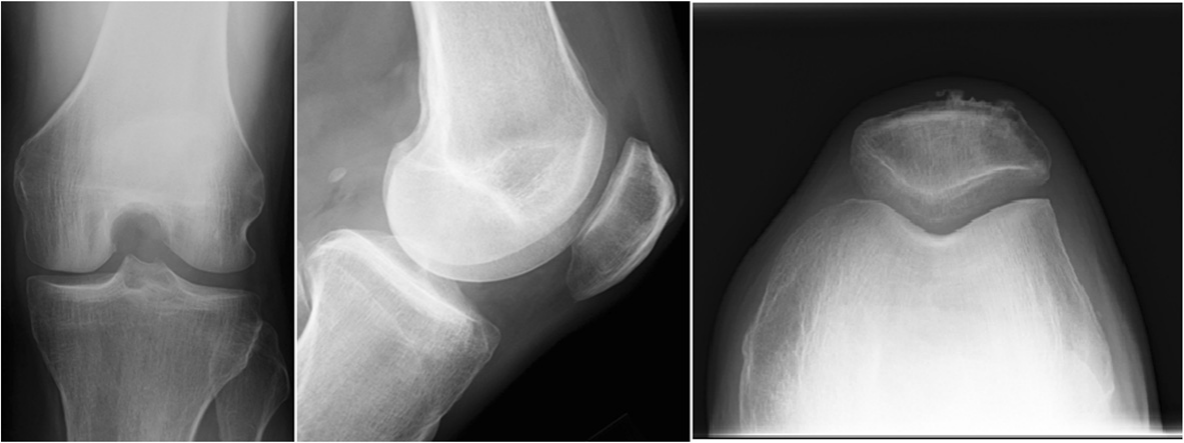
AP, lateral, and sunrise radiographs of the knee demonstrate a lucent lesion of the superolateral patella.
